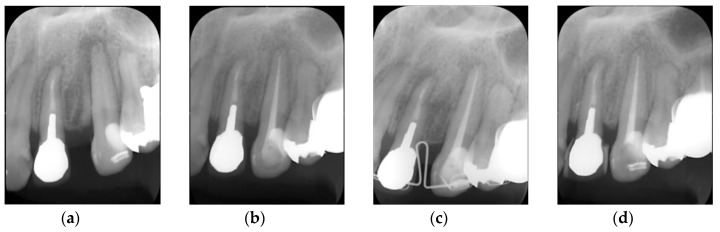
Clinical performance of CS-BG. (a) Pre-treatment radiograph. (b) Post-obturation radiograph. (c) 6 months follow-up radiograph. (d) 14 months follow-up radiograph.
